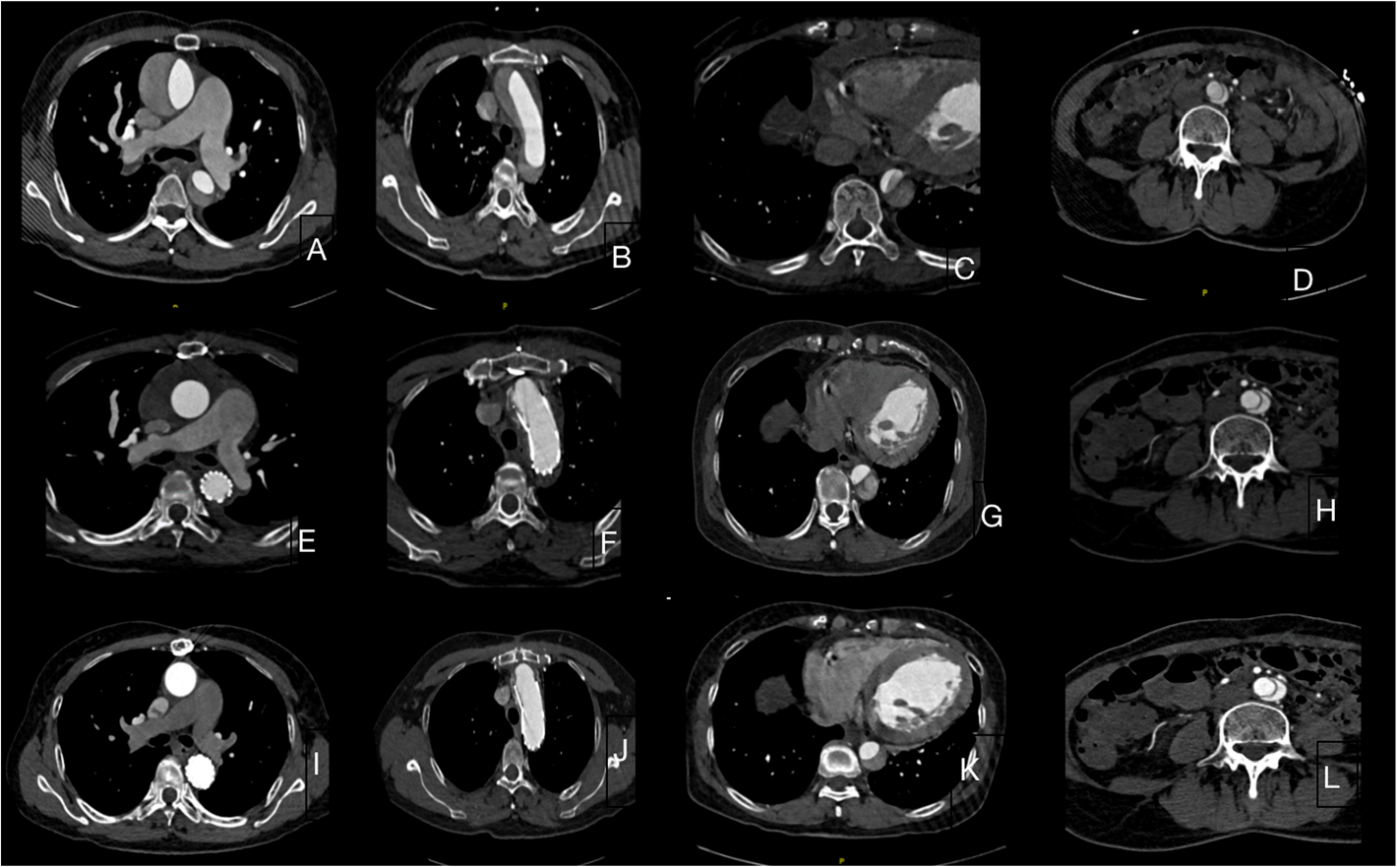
CTA about the aorta at different levels at admission,before discharge, and 3 months after discharge. The upper four pictures stands for the aorta at the bifurcation of the pulmonary artery, aortic arch, and diaphragm and at the bifurcation of the common iliac artery when admitted in hospital (a-d). The middle and final four pictures stands for the aorta at the bifurcation of the pulmonary artery, aortic arch,and diaphragm and at the bifurcation of the common iliac artery when before discharge(e-h) and 3 months after discharge(i-l)
